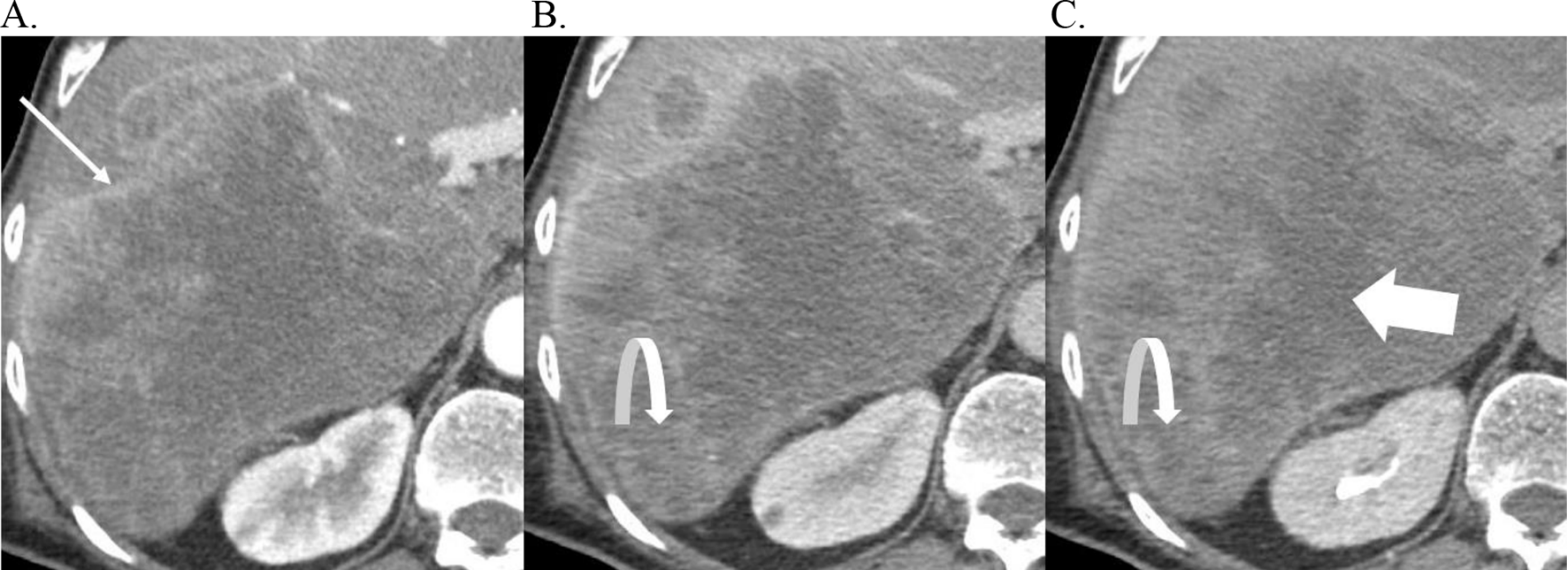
Necrosis. Axial contrast enhanced CT in the arterial phase (A), portal venous phase (B), and delayed phase (C), demonstrating a pathologically proven necrotic CCA with rim APHE (arrow), delayed central enhancement (curved arrow), and non-enhancing necrosis centrally (large arrow)
